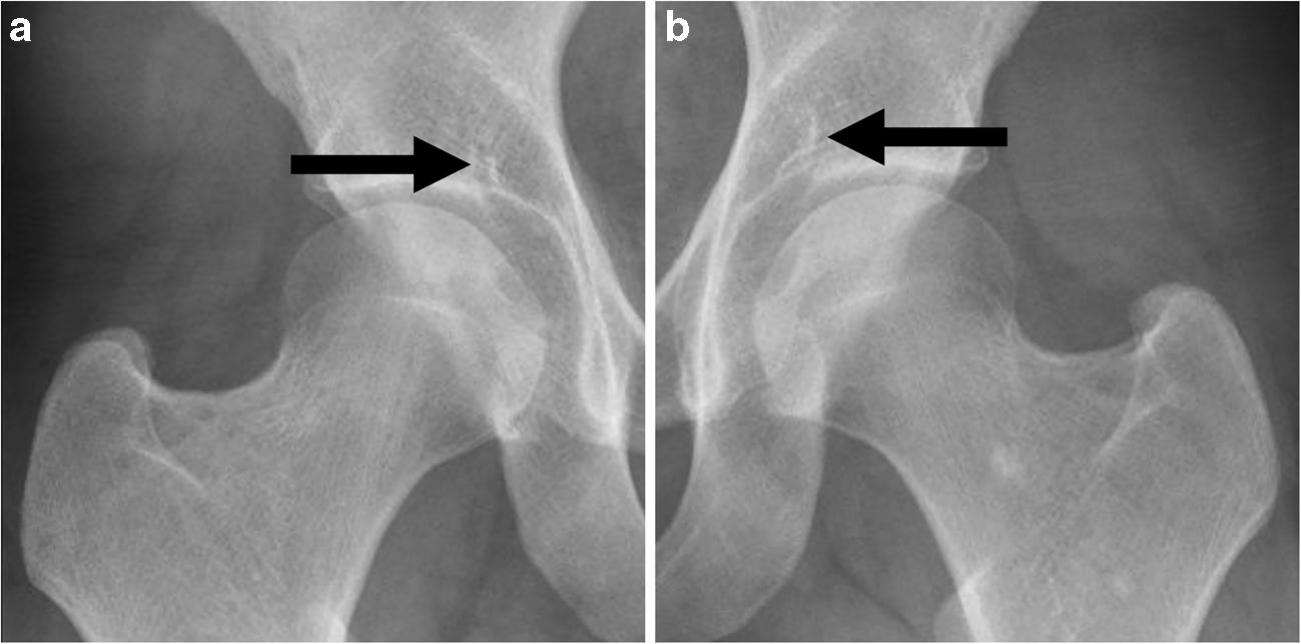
Twenty-four-year-old female. Anteroposterior radiographs show bilateral superior acetabular roof notches (SARN) (arrows) (a right hip; b, left hip). The SARN was originally described as “a radiologic discontinuity in the medial aspect of the acetabular roof” on radiographs [3]
